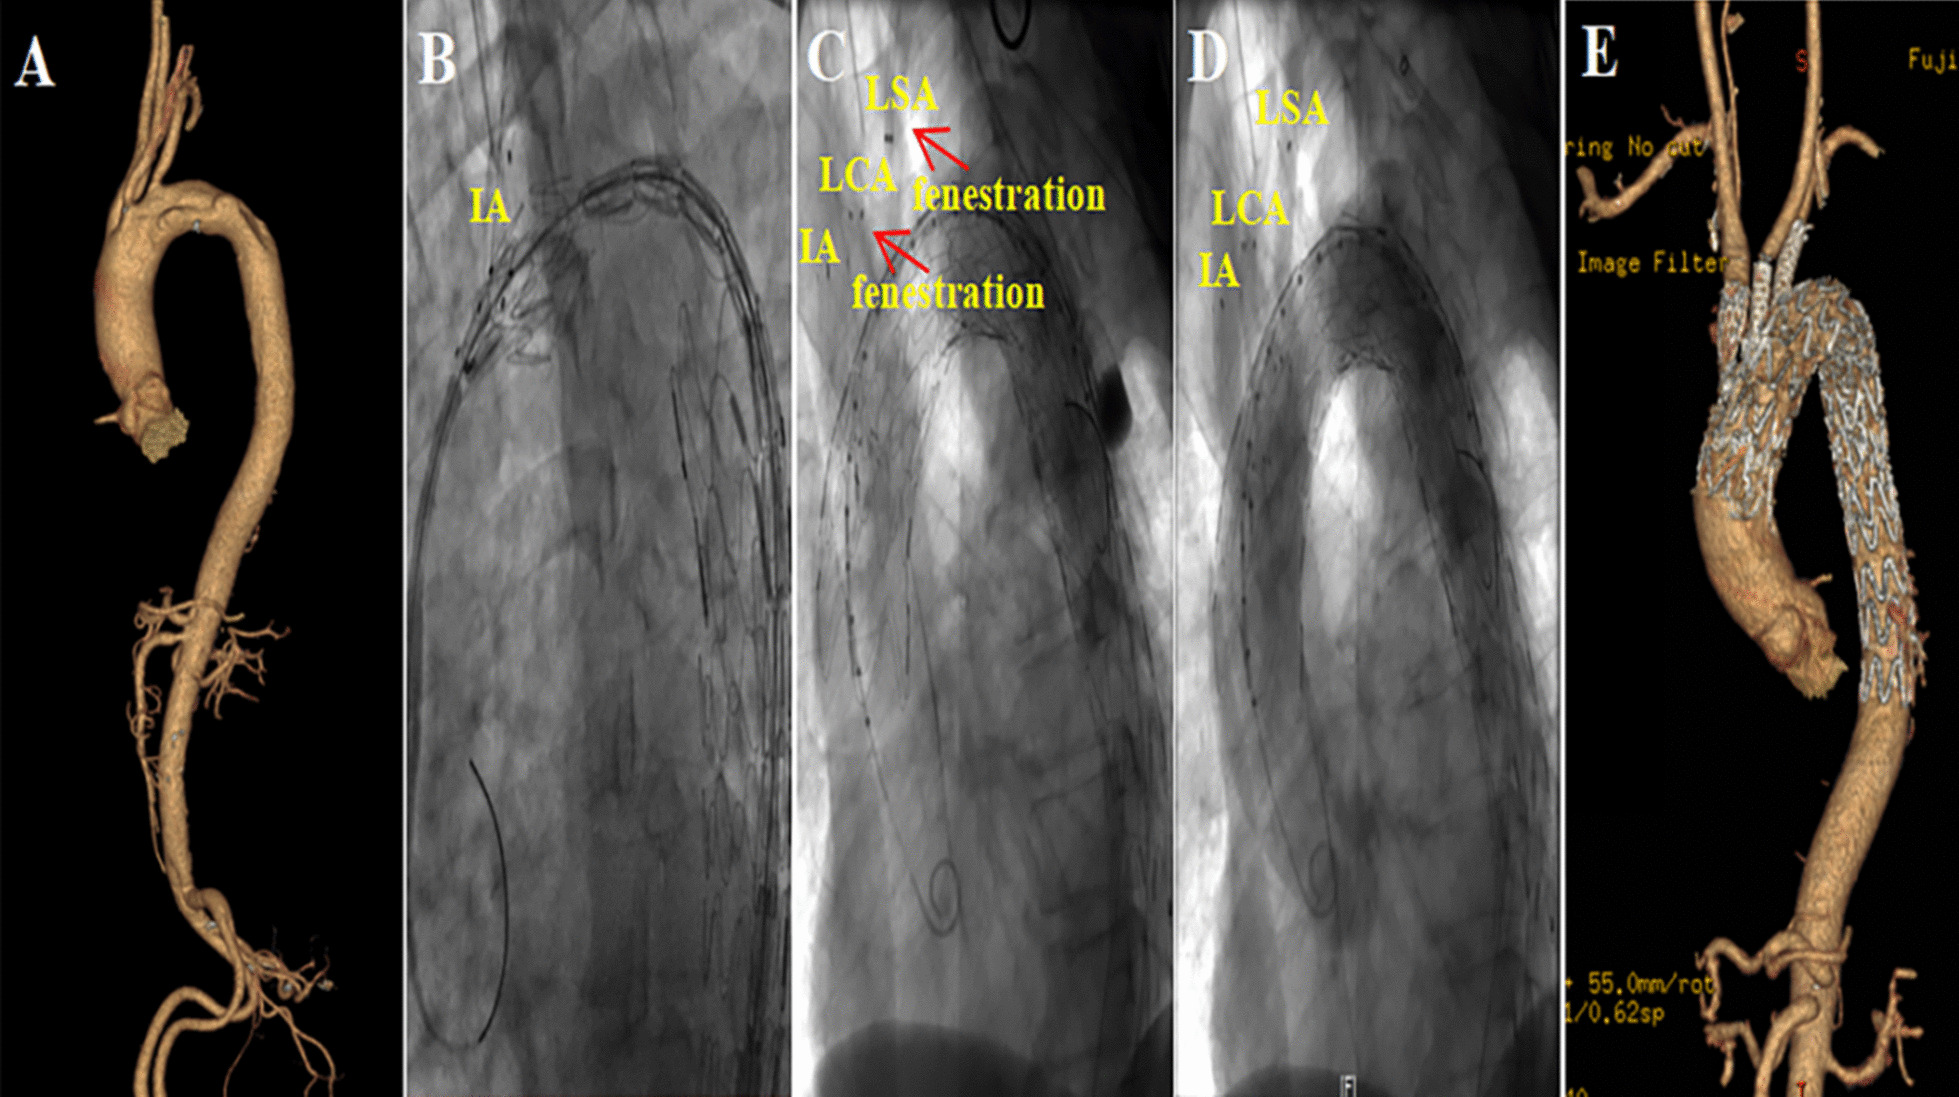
Total reconstruction of supra-aortic branches. A CTA display aortic pathologies involving the aortic arch. B The single-branch of “Castor” was pulled into the IA along the right IA track. C A covered short self-expanding stent graft of appropriate size was implanted and deployed in the in-vitro fenestration of LCA and LSA, respectively. D DSA show the perfect patency of supra-aortic branches. E Postoperative CTA show the perfect patency of the supra-aortic branches
